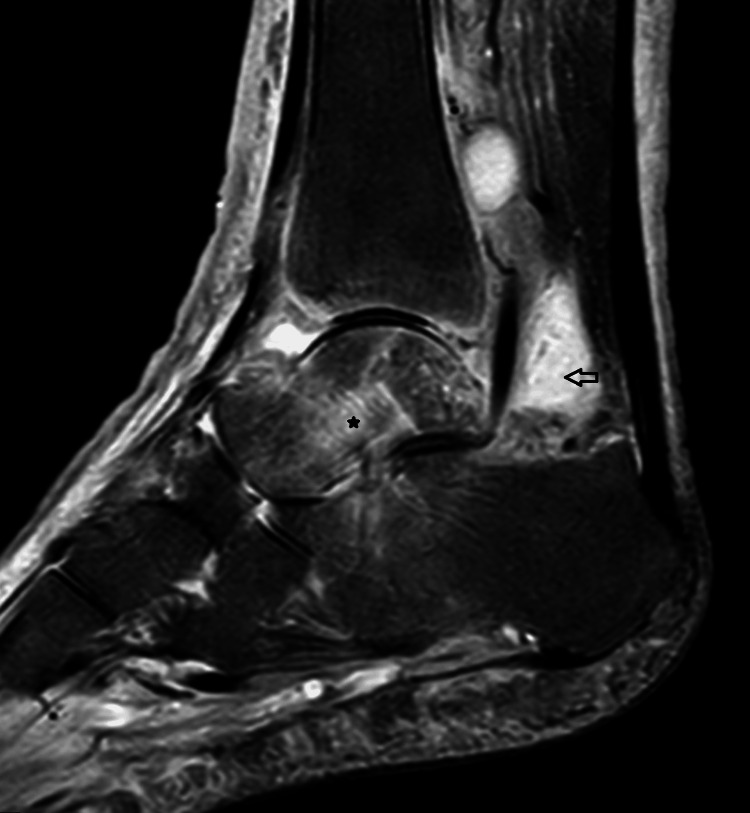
MRI of the left ankle (sagittal STIR sequence). Mixed hyperintense signals within the synovial membrane and joint cavity (blank arrow), indicating the presence of inflammation and increased effusion typical of synovitis, which appears extensive and severe in this case. Reactive marrow oedema is also noted (asterisk).
